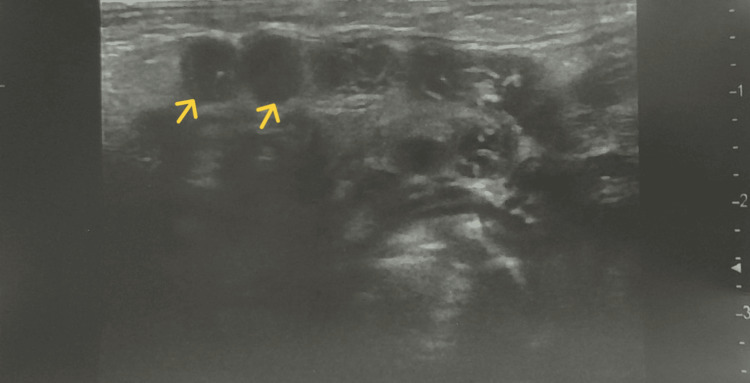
Ultrasound of the mentonian mass showing digestive structures (arrows) consistent with a parasitic twin
